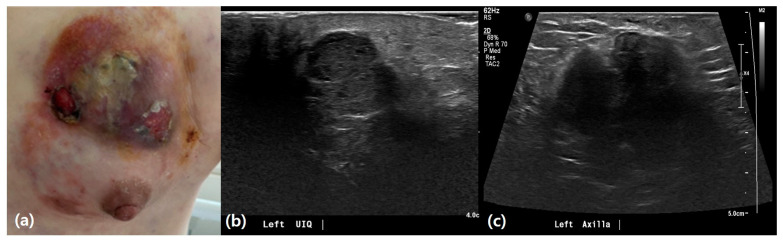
Inflammatory breast cancer. A 40-year-old female patient presented with a palpable mass and skin changes in her left breast. (a) Clinical appearance showing erythema, skin thickening, and ulcerative changes at the time of the visit. (b) Ultrasound imaging demonstrates an irregular hypoechoic mass with diffuse skin thickening and edematous changes, primarily in the left upper inner quadrant. (c) Ultrasound imaging also shows a conglomerated lymph node, approximately 4.7 cm in size, in the left axillary level I, suggestive of metastatic involvement.
